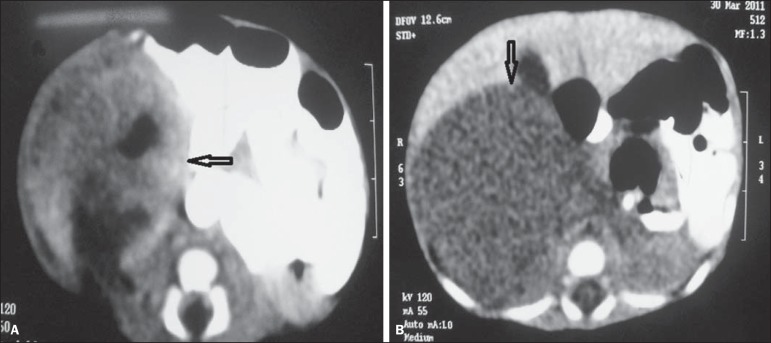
Abdominal computed tomography. A: Transverse section
